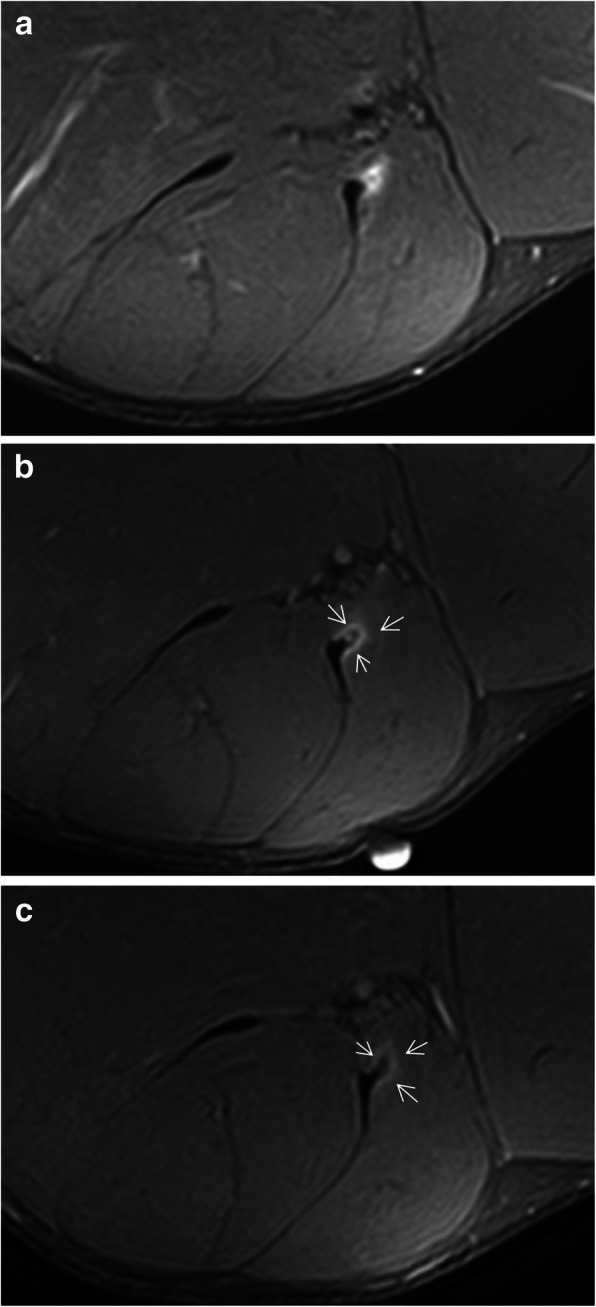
Evolution of healing by MRI over a course of 15 days: hamstring myotendinous junction. Axial fluid-sensitive images at 24 h (a), 8 days (b), and 15 days (c). At 8 days, the soft scar is present with centripetal growth although with reparative pericicatricial edema that remains at 15 days (b and c, arrows)
